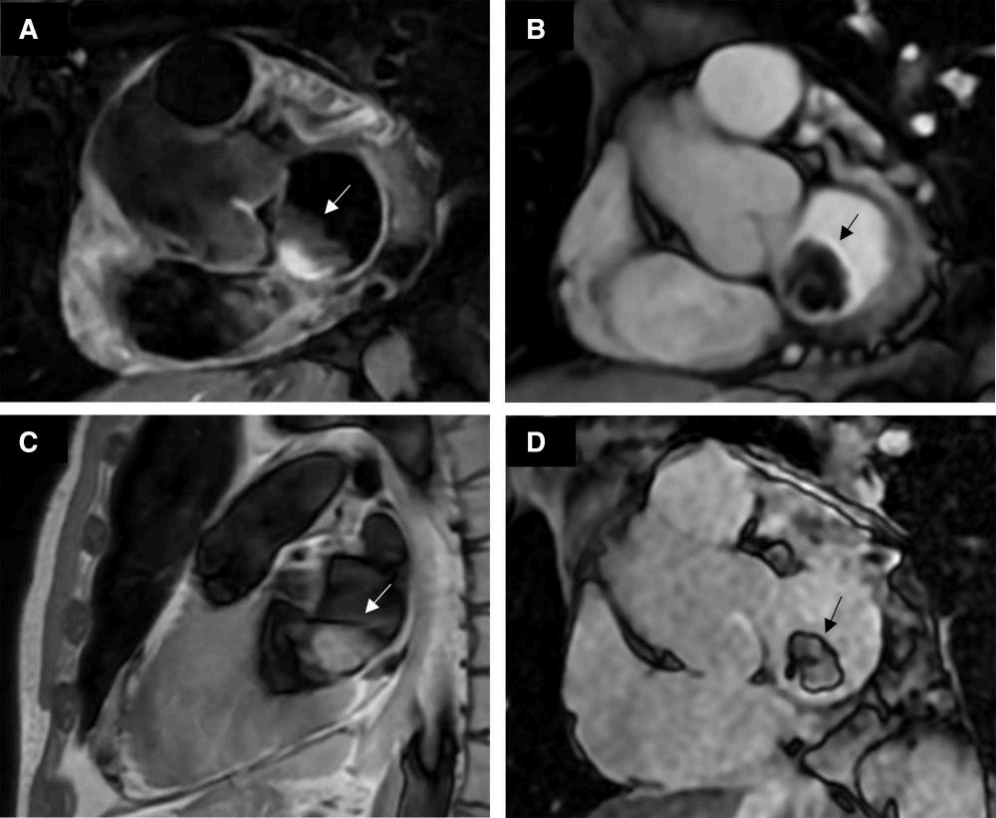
CMR, left atrial mass. CMR T2-weighted black-blood image (*A*), cine SSFP image (*B*), T1-weighted image (*C*), and myocardial delayed enhancement image (*D*) showing the left atrial mass suspected to be a left atrial myxoma.
